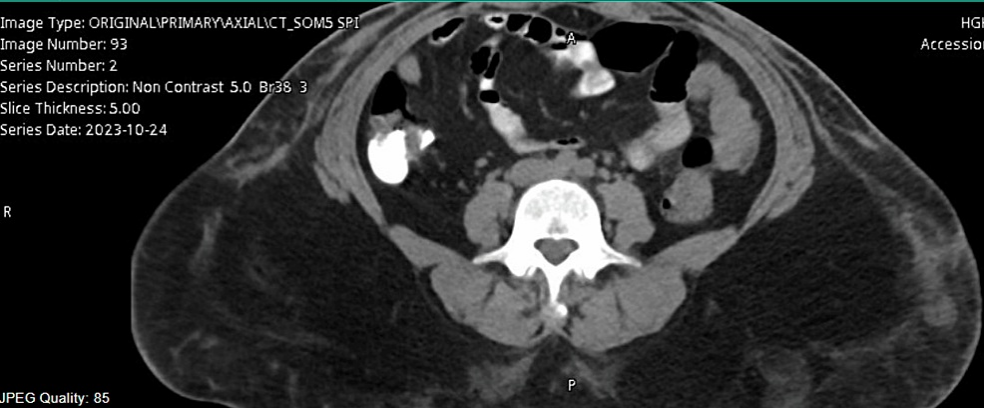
CT at the first visit to ED showing subcutaneous tissue swelling, debris accumulation
